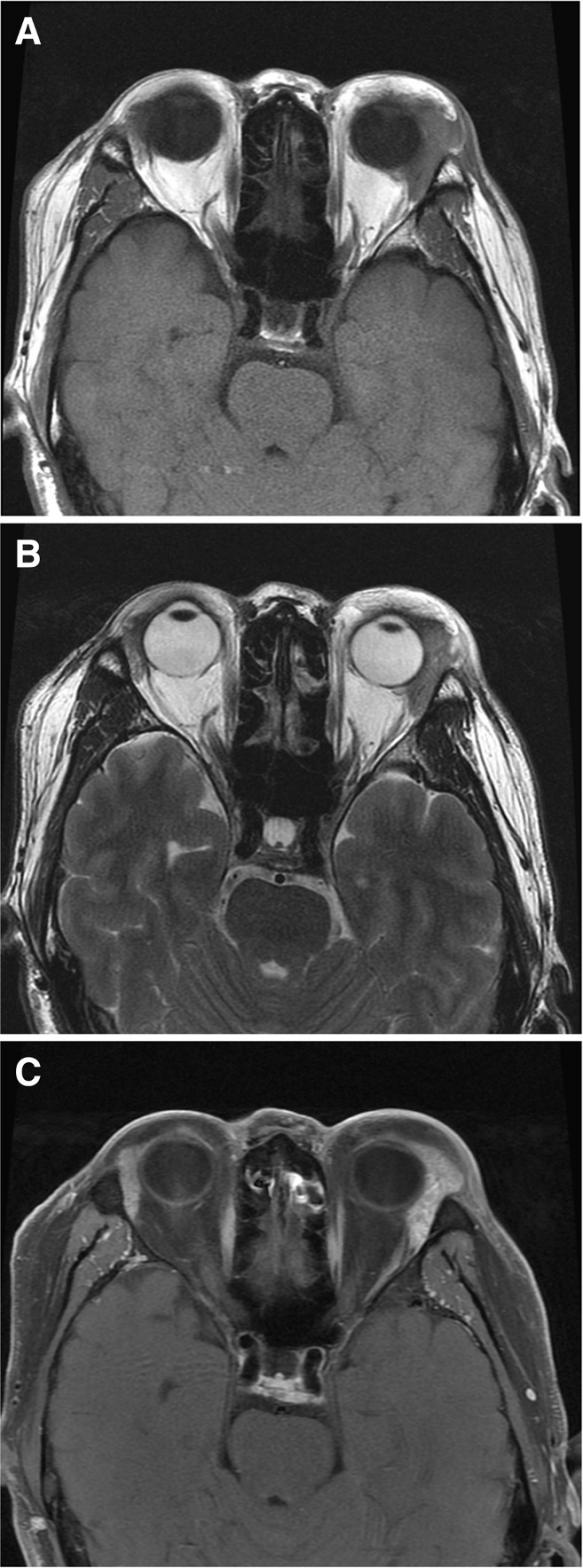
Magnetic resonance imaging (MRI) examination of the orbit showed that the left lacrimal gland was lobulated lobulated with a distinct margin and was predominantly isointense on T1-weighted images (A), isointense on T2-weighted images (B) and an obvious heterogeneous enhancement on contrast-enhanced MRI images (C).
